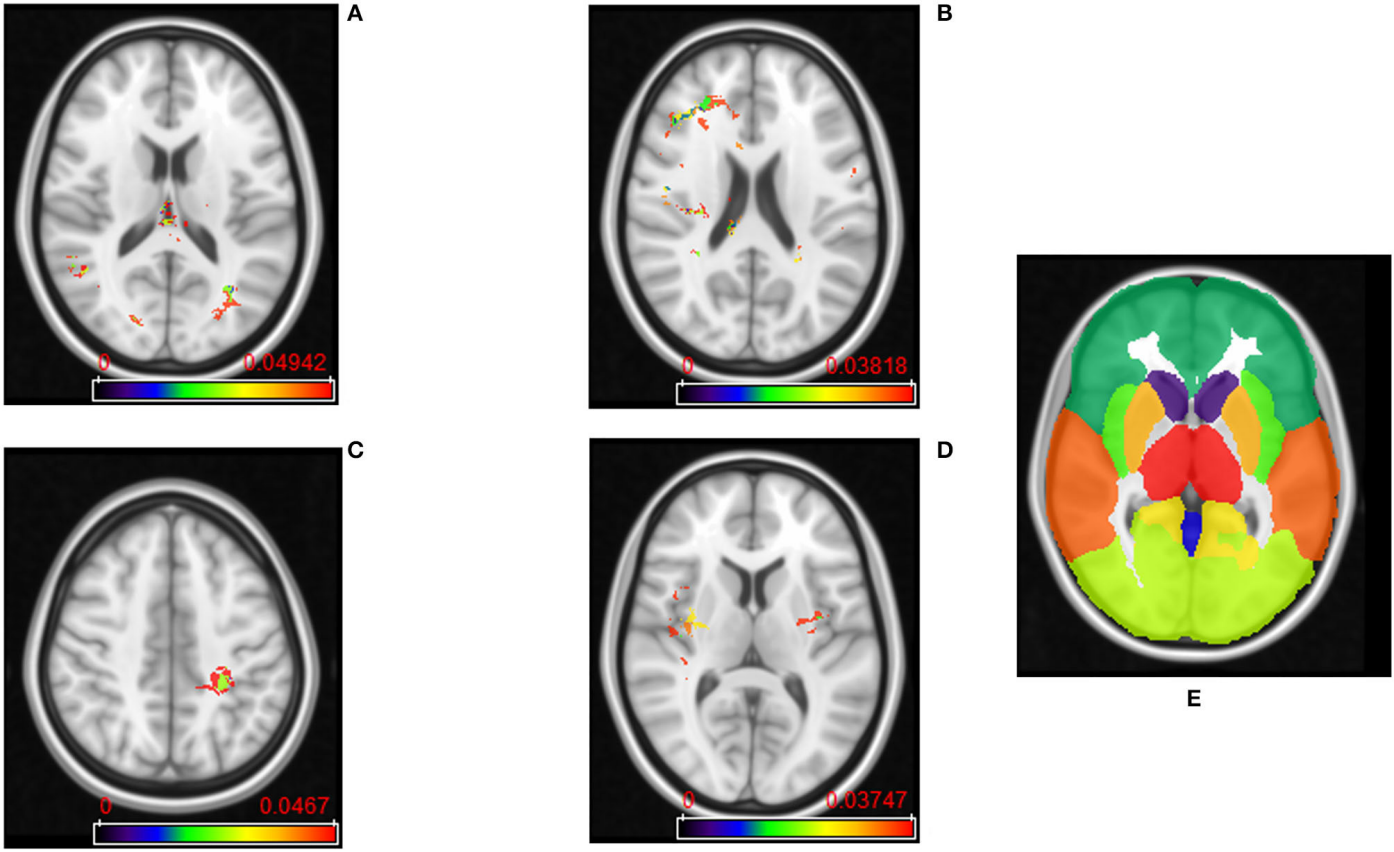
(A) ADIFFI maps for peri-lesional T2/FLAIR hyperintensities in tumor recurrence, and (B) pseudo-progression. (C) ADIFFI maps for enhancing lesion in tumor recurrence, and (D) pseudo-progression. The level of significance was at a *p*-value of 0.05 for all of these maps. These were the maps prior to applying RP analysis. (E) The labeled anatomical MNI atlas that is used for parcellating ADIFFI maps and identifying significant areas.
